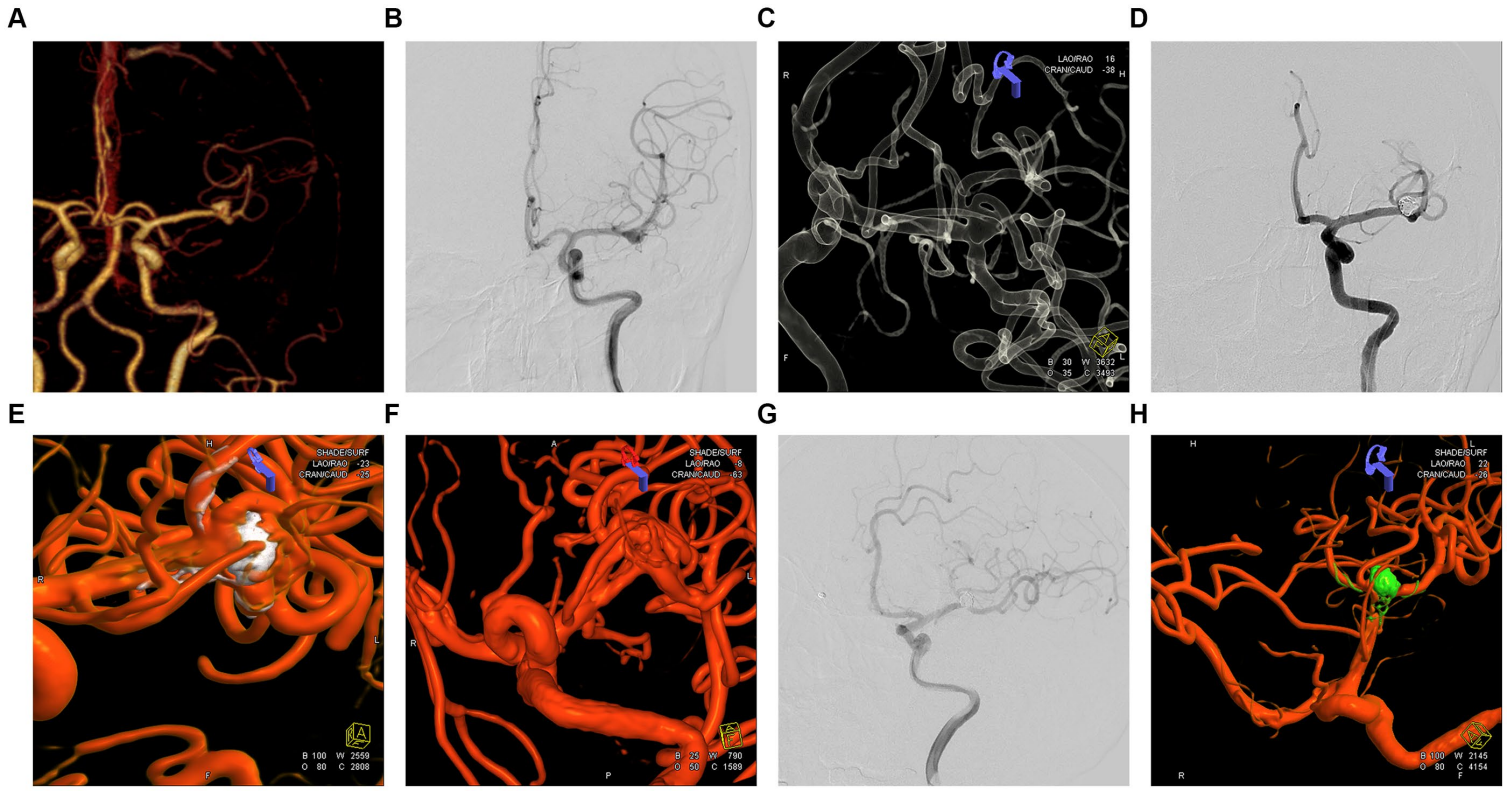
Embolization and imaging follow-up of an unruptured left middle cerebral artery (MCA) bifurcation aneurysm. (A) A 57-year-old woman was admitted for treatment of a left middle cerebral artery aneurysm diagnosed by CTA. (B) Orthostatic cerebral angiography revealed a wide-necked bifurcation aneurysm in the left MCA. (C) 3D reconstruction showing the left MCA aneurysm involving three middle cerebral artery branches, and Y-stent-assisted embolization was proposed for treating the aneurysm. (D) Postoperative 3D angiography showing embolization of the aneurysm in the left MCA bifurcation. (E,F) The 3D reconstruction reveals the embolization status of the aneurysm at the bifurcation of the left MCA. The embolization result is classified as mRRC III b, with partial opacification observed in the aneurysmal sac. (G,H) Postoperative orthopantomography and 3D reconstruction demonstrating improvement in the embolization of the aneurysm located in the branch of the left MCA at the seventh month after surgery, with the aneurysm completely and densely embolized to mRRC I.
